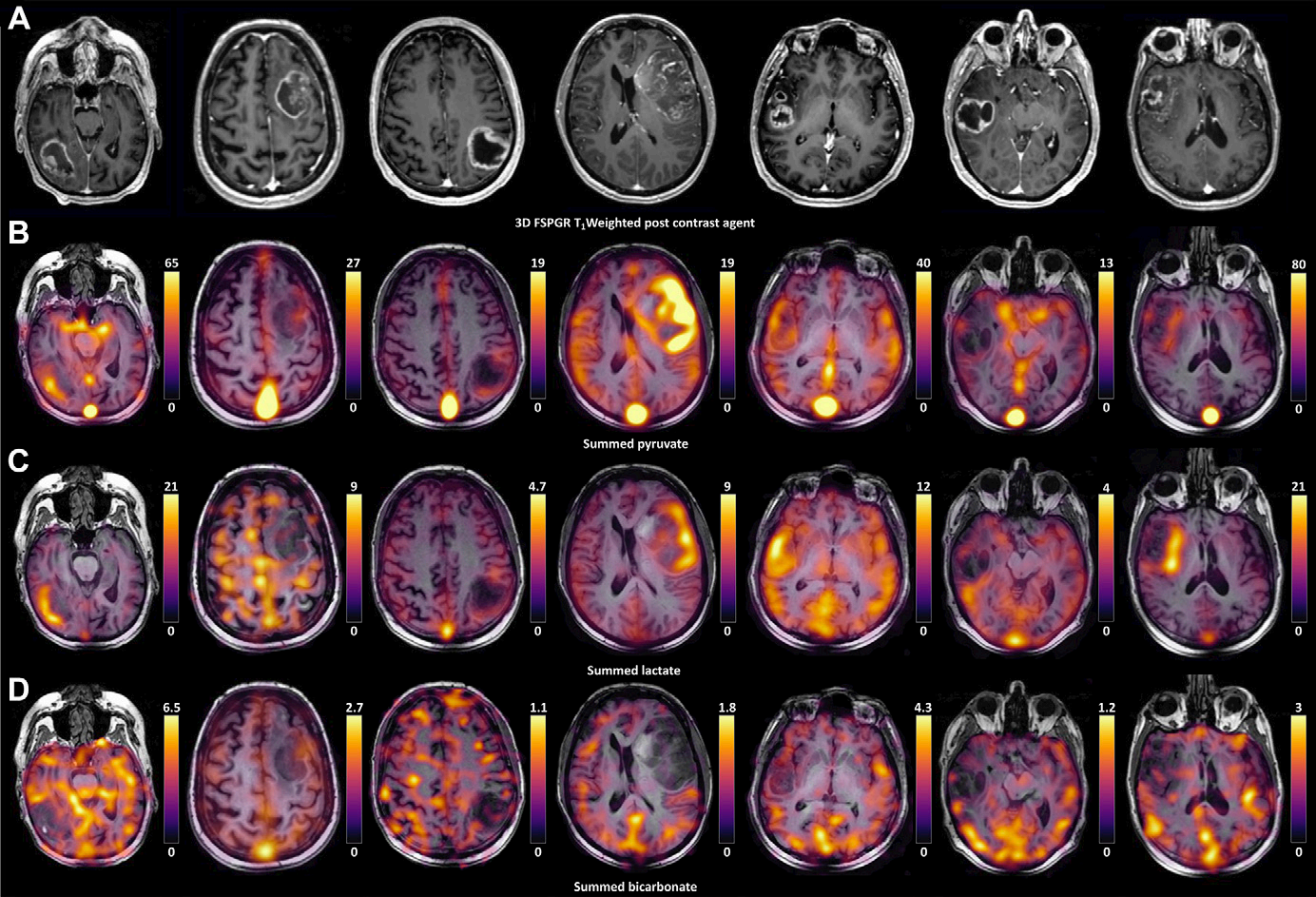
Hyperpolarized ^13^C MR images from all seven patients.
